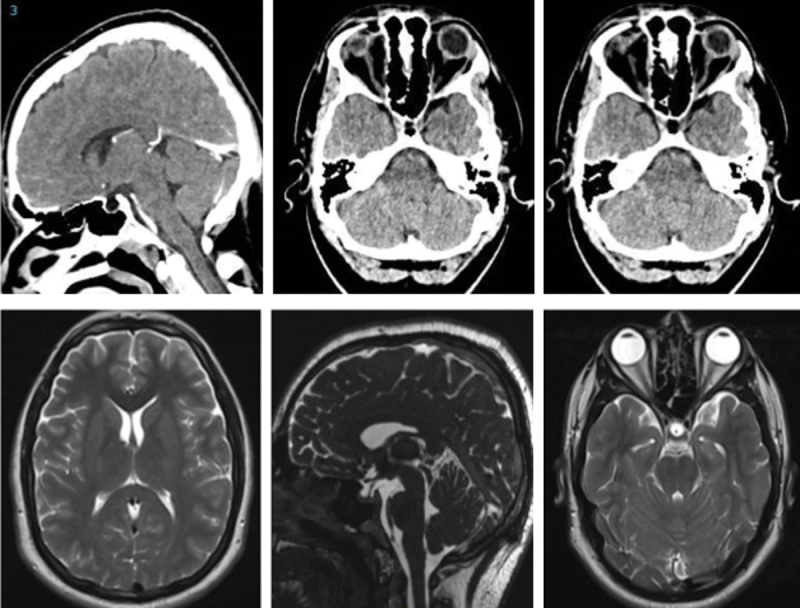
Computed tomography scans (upper row) and magnetic resonance imaging (lower row) showing a partially empty sella turcica and small ventricles, suggestive of intracranial hypertension.
